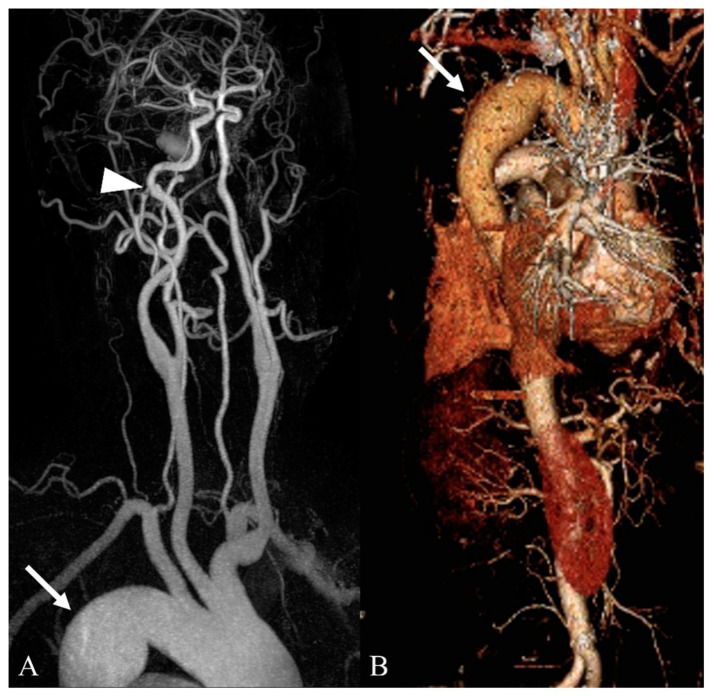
Incidentally detected aortic aneurysm during work-up for a headache in a patient with renal cell carcinoma. (A) In contrast-enhanced neck MR angiography (3D MIP image), there is a saccular aneurysm in the distal aortic arch (arrow). In the left distal internal carotid artery (C1 segment), a small bulging lesion is noted with a flap-like lesion, suggesting focal dissection (arrowhead). (B) In contrast-enhanced aorta CT, the aortic aneurysm is clearly depicted (arrow).
